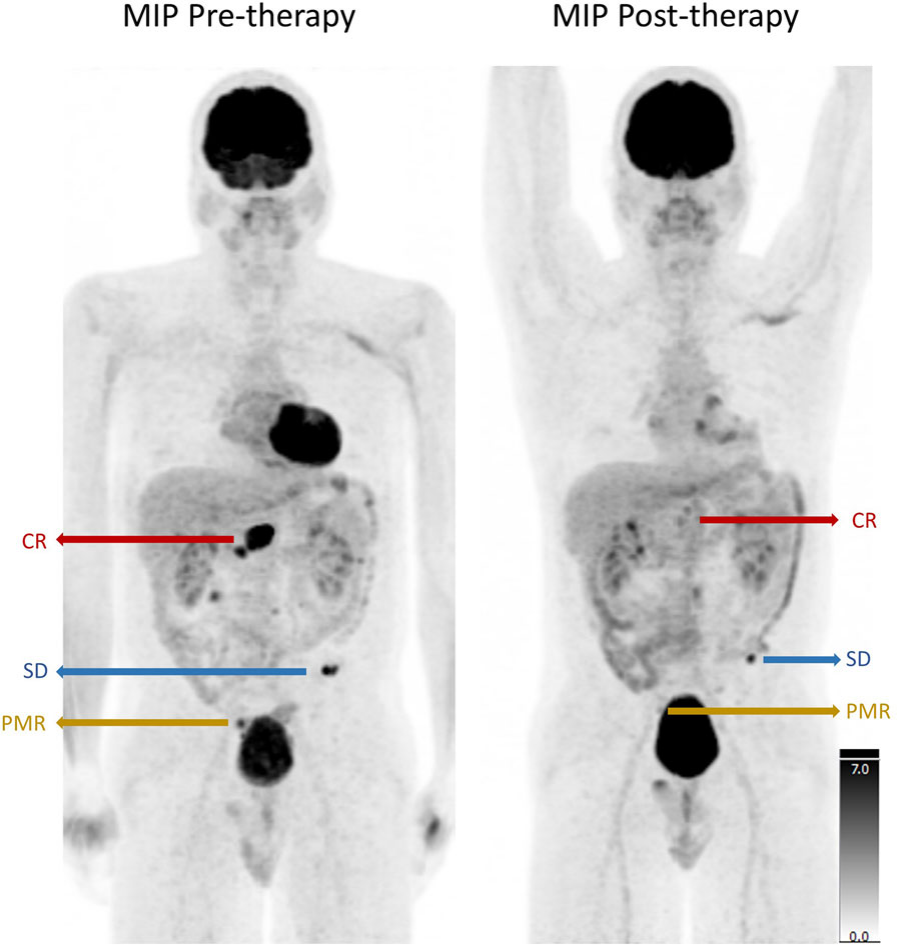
Maximal intensity projection (MIP) images of the ^18^F–FDG-PET scan at baseline and after regorafenib treatment. CR = complete response; SD = stable disease; Deep PMR = deep partial metabolic response
